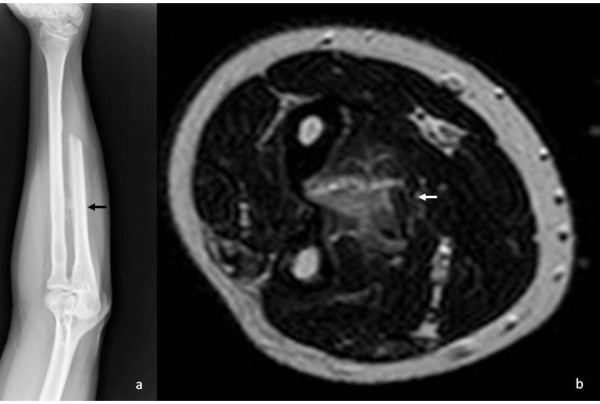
After 18 months X-rays showed small ossifying mass in the interosseus membrane. It is not clear if there was contact with the bone (a); MRI confirmed the presence of the mass that did not seem to involve the ulna or the radius (b).
